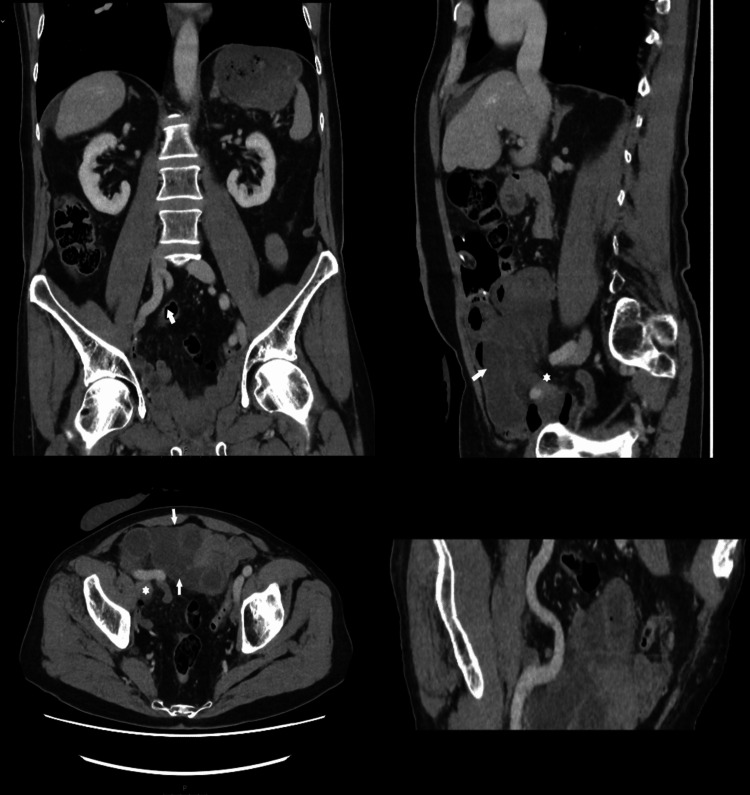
Computerized tomography (CT) images show that the right external iliac artery takes an abnormal medial turn after bifurcation of the right common iliac. This is denoted by the arrow in the top-left coronal film and more accurately in the reconstructed angulated film at the bottom-right. Axial film (bottom-left) shows strangulated bowel loops (arrows) herniating underneath the abnormal right external iliac artery. Loops proximal to the herniation point can also be seen here (asterisk). The sagittal film in the top-right shows the herniation point (asterisk) and the herniated bowel loops (arrow).
